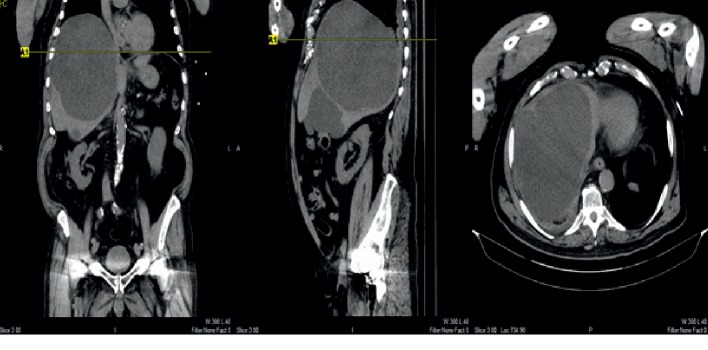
Initial CT abdomen/pelvis with multiple views of large hepatic cysts.
